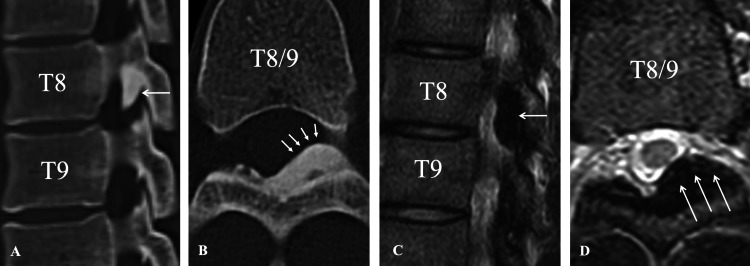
Preoperative CT and MRI images demonstrating left-sided ossification of the ligamentum flavum at T8-T9 A) Sagittal CT image showing localized hypertrophic ossification of the ligamentum flavum and foraminal narrowing at the T8-T9 level (arrow); B) Axial CT revealing localized ossification at the T8-T9 level (arrows); C) Sagittal T2-weighted MRI showing localized hypertrophic ossification of the ligamentum flavum and foraminal narrowing at the T8-T9 level (arrow); D) Axial T2-weighted MRI showing left-sided foraminal stenosis without spinal cord compression (arrows) CT - computed tomography; MRI - magnetic resonance imaging
